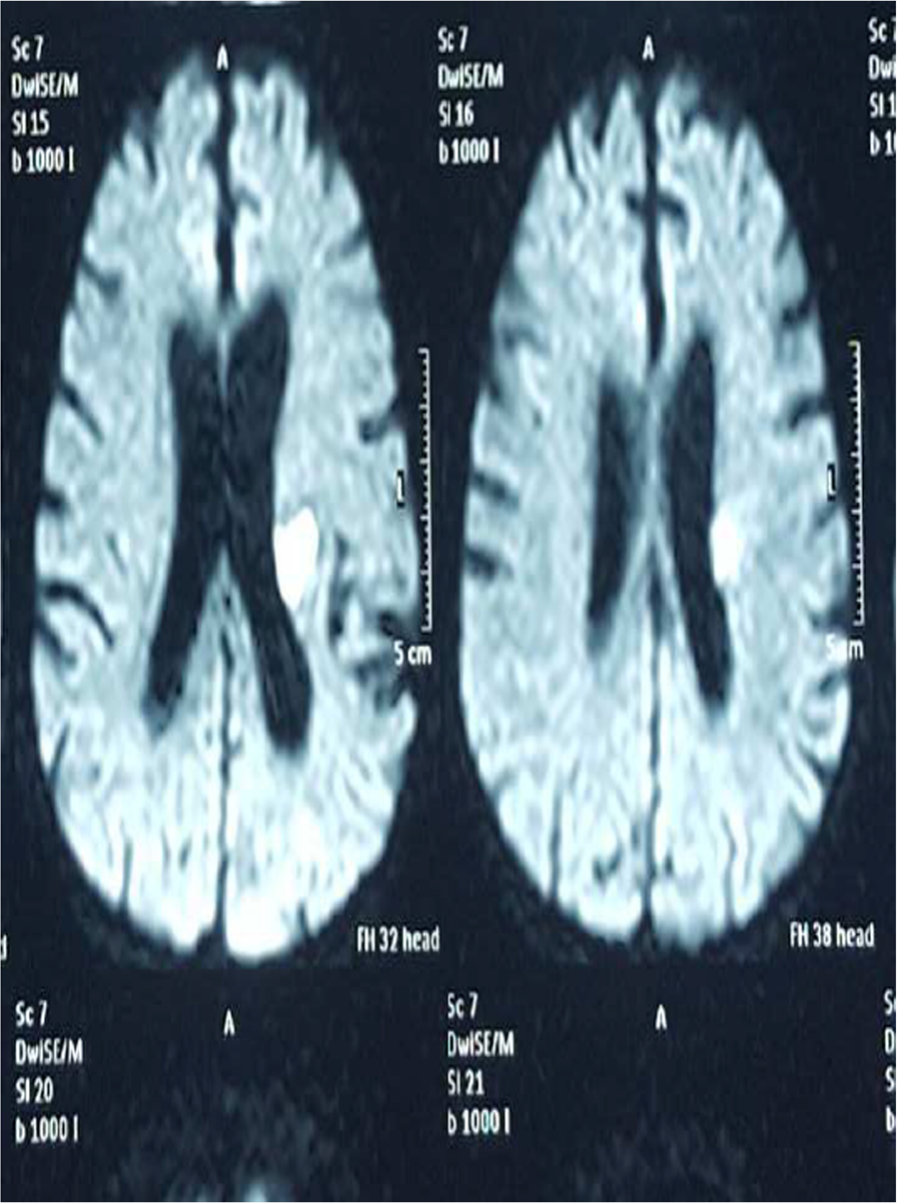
Magnetic resonance imaging showing an acute infarction in the left posterior frontal white matter
